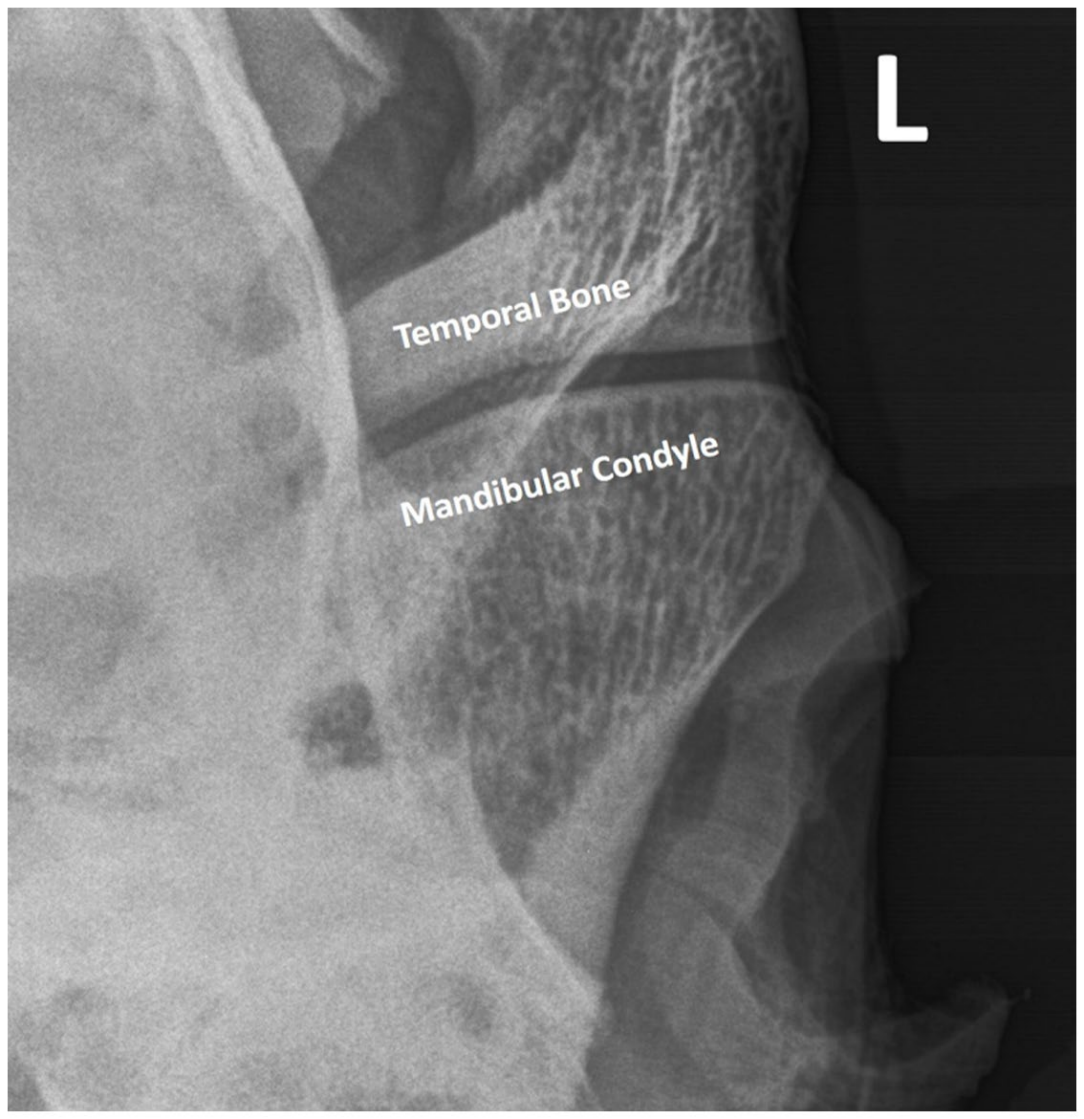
The radiographic image obtained using the R45°V30°L-CdDLO projection. The intra-articular disc is not delineated but is present between the temporal bone and mandibular condyle.
